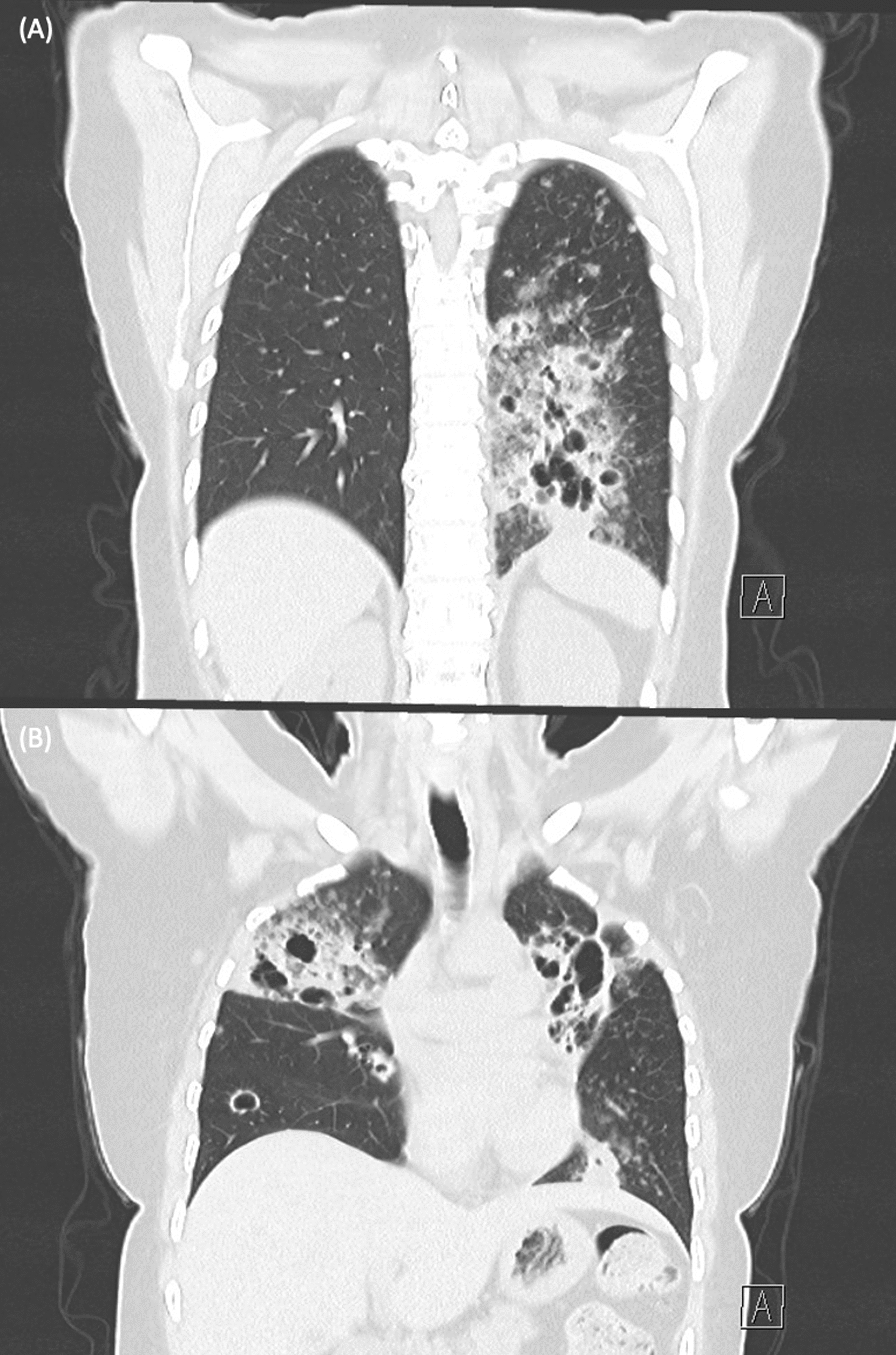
A, B Coronal view of computed tomography imaging showing multilobar cavitary lesions and extensive centrilobular ground-glass nodules
